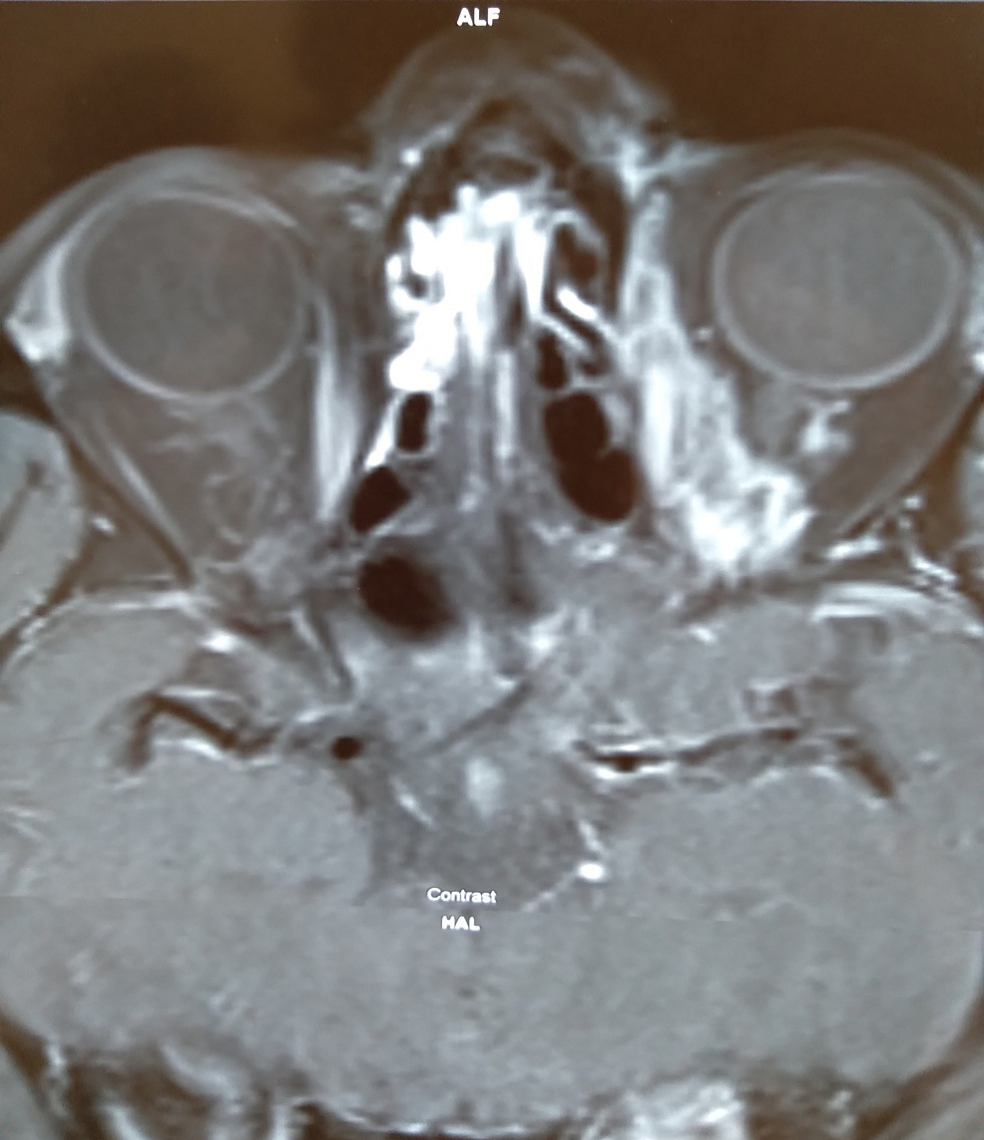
MRI of orbit revealed swollen left optic nerve and capillary hemangioma in the retrobulbar region MRI: magnetic resonance imaging
